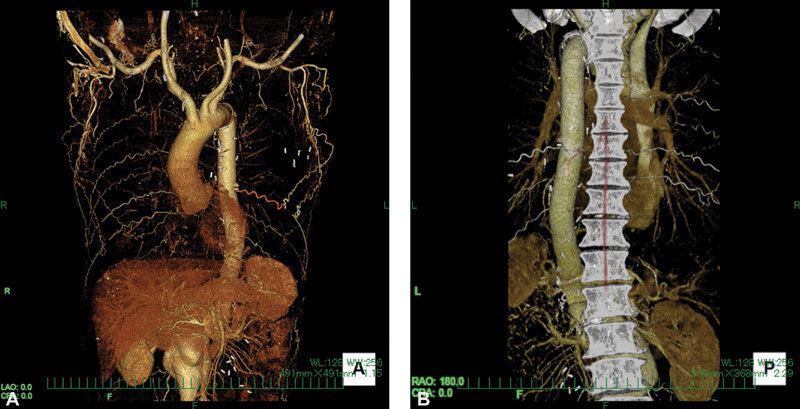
Preoperative computed tomography showing a collateral vessel from the left lateral thoracic artery to the Adamkiewicz artery. (
